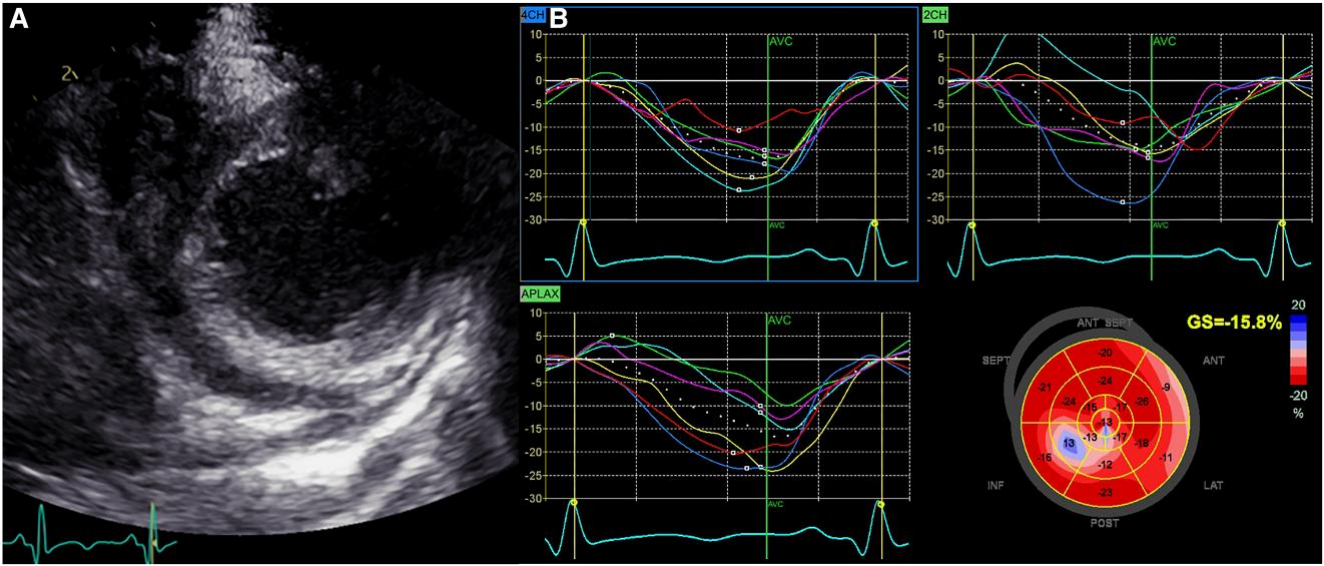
Standard echocardiography and speckle-tracking analysis in paediatric myocarditis. (*A*) Parasternal short-axis view of the left ventricle on 2D echocardiography. 2D parasternal short-axis view of the left ventricle showing segmental hyper-echogenicity in the inferior wall. (*B*) Speckle-tracking assessment of left ventricular global longitudinal strain (GLS) in a child with myocarditis. Speckle-tracking analysis with a bull’s-eye plot for the same patient reveals a reduced global longitudinal strain (−15.8%) and pronounced segmental abnormalities in the inferior segments of the left ventricle.
